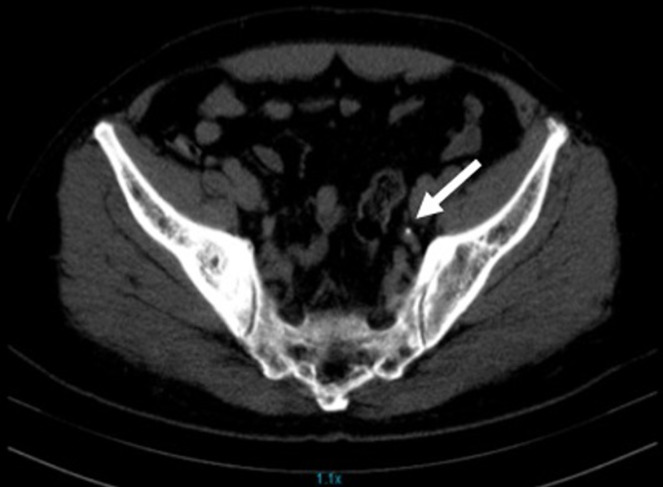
computed tomography of kidneys, ureters, and bladder revealed an obstructive left ureteric calculus at the vesicoureteric junction (VUJ) measuring 4.7 mm with no obvious fat stranding
